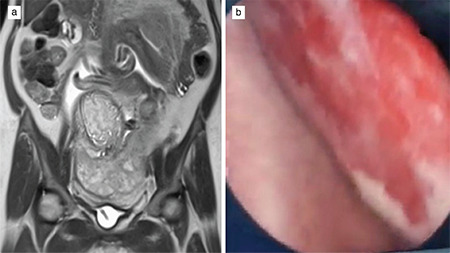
(a) T2 weighted magnetic resonance imaging (coronal section) showing placenta invading through the lower uterine segment reaching up to bladder serosa; (b) Cystoscopy showing placental bulge with intact bladder mucosa
